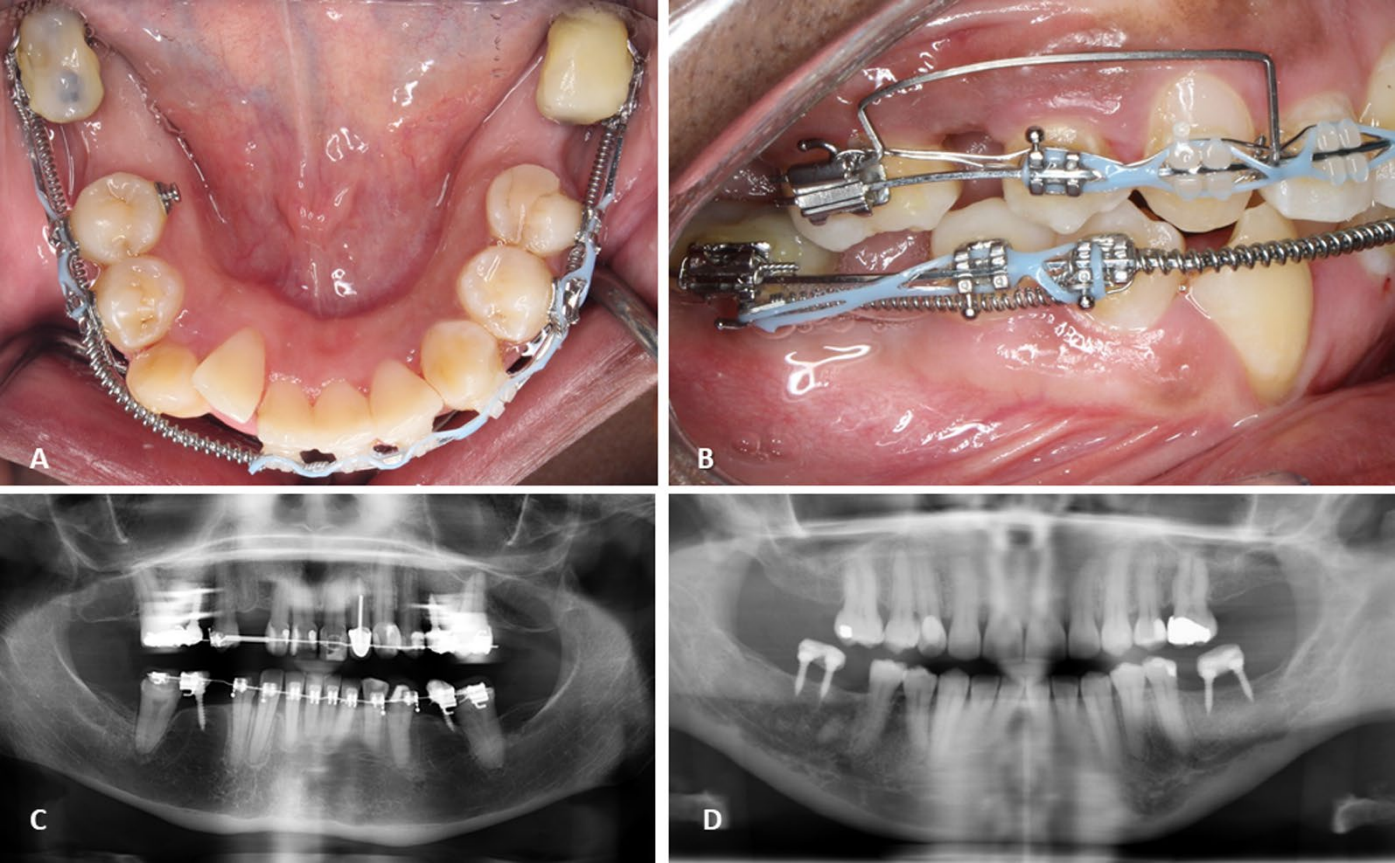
Ridge mini-implants clinical and radiographic examples. A Splinted ridge mini-implants in the mandibular edentulous region. B Lateral view of the splinted mini-implants used for retraction of the premolar region. C Panoramic radiograph depicting single mini-implants, one on each quadrant. D Panoramic radiograph of clinical photograph in A and B showing splinted mini-implants
